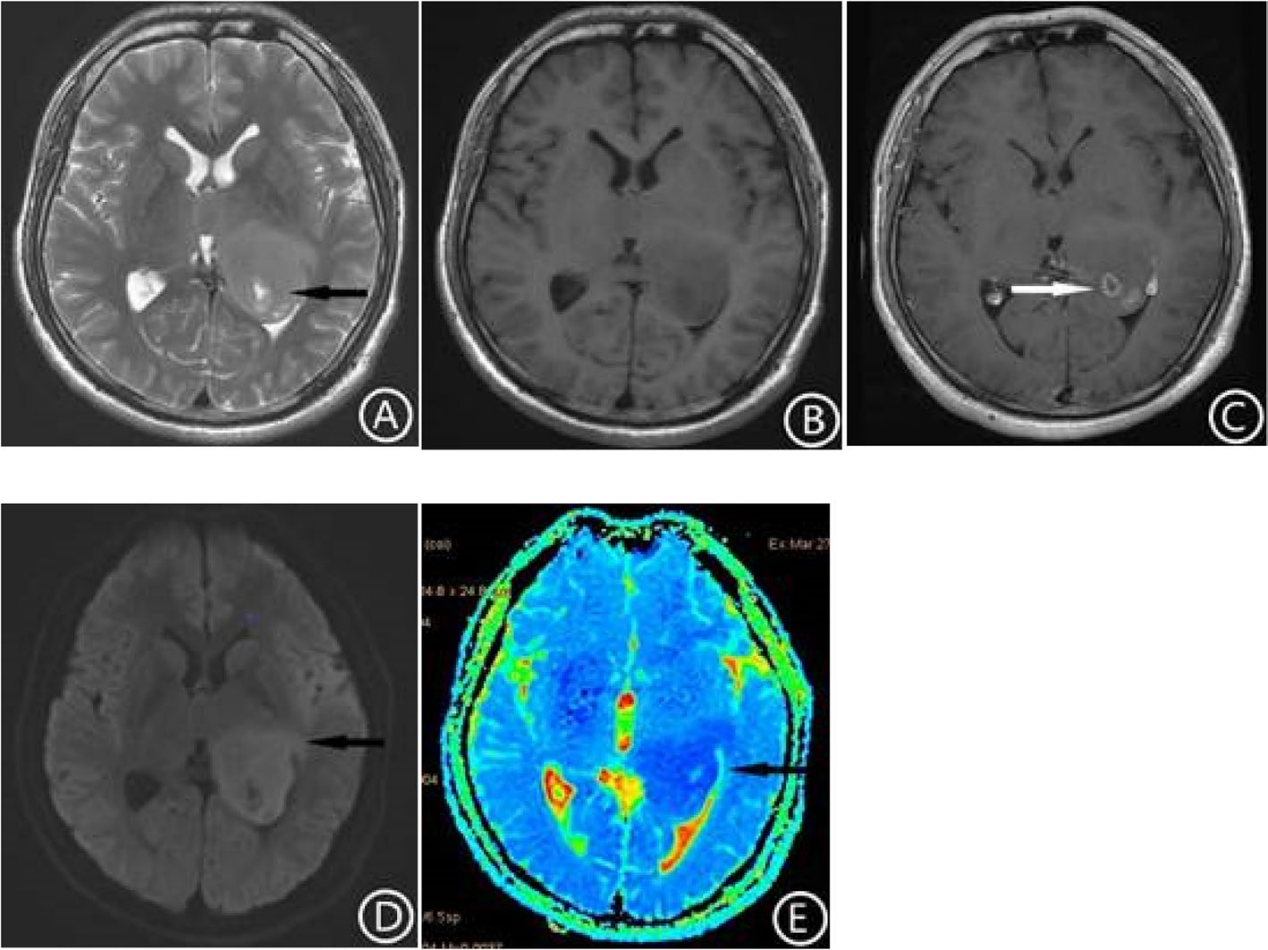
Brain MRI, DWI and ADC mapping in patients with Diffuse midline glioma, H3 K27-altered. The lesion was located in the left thalamus and invaded the left ventricle. The internal signal of the tumor was uneven, T2WI showed high signal intensity (A), and T1WI showed low signal intensity (B). Multiple cysts can be seen in the part of the tumor invading the lateral ventricle (A, arrow). There was no edema around the lesion. After enhancement, the tumor showed inhomogeneous enhancement, with circular enhancement (C, arrow). The tumor tissue showed high signal intensity on DWI. The boundary of the tumor was unclear and grew infiltratively along the lateral ventricular wall (D, arrow). On ADC mapping, the lesion showed low signal (E). The ADC value of the tumor tissue (6.97 × 10^− 4^ mm^2^/s) was significantly lower than that of the contralateral side (8.47 × 10^− 4^ mm^2^/s)
